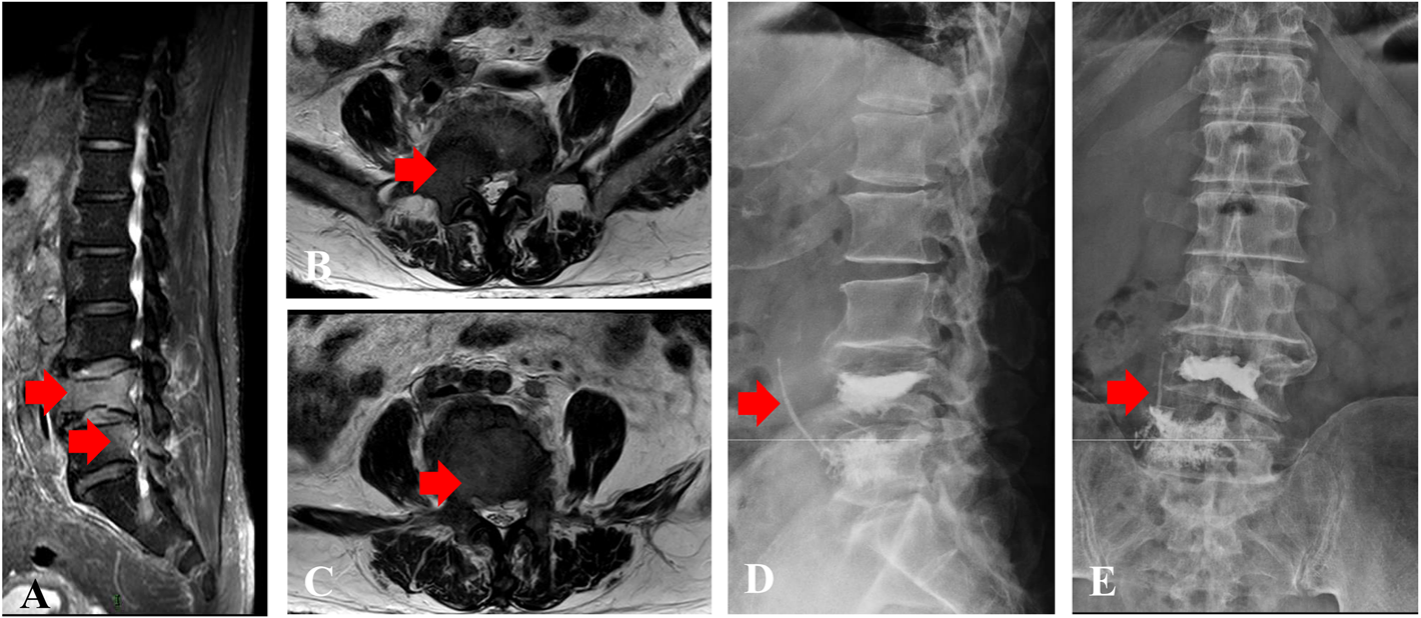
An 80-year-old female with spine metastases treated with percutaneous vertebroplasty. A Preoperative sagittal lumbar vertebra MRI (T2) showed spine metastases at L4 and L5; B Preoperative transversal MRI (T2) showed metastatic lesion at L4; C. Preoperative transversal MRI (T2) showed metastatic lesion at L5; The red arrow indicates metastatic tumor in (A, B, and C). D Postoperative lateral lumbar vertebra X-ray presented bone cement at L4 and L5 and vascular cement leakage; E Postoperative anteroposterior lumbar vertebra X-ray presented bone cement at L4 and L5 and vascular cement leakage. The red arrow indicates vascular cement leakage in (D and E)
